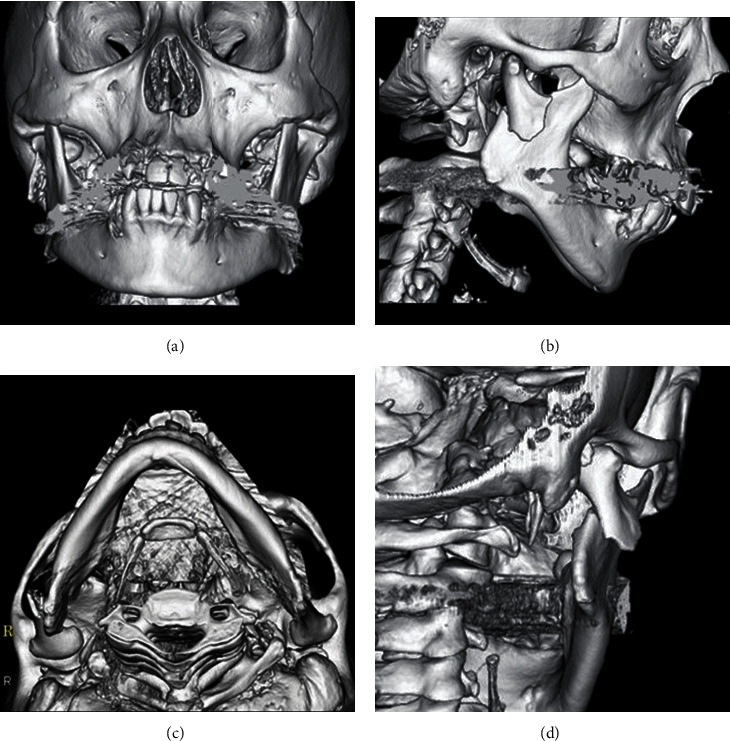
3D-constructed CT image at the first visit. (a) Front. (b) Right side. (c) Axial. (d) 45 degrees behind to the right. CT imaging shows a right mandibular condylar base fracture and lateral dislocation of the fracture fragment. No fractures are observed except for those of the mandible. No fractures of the alveolar bones of the upper and lower jaws are also observed. No tooth fracture is observed.
